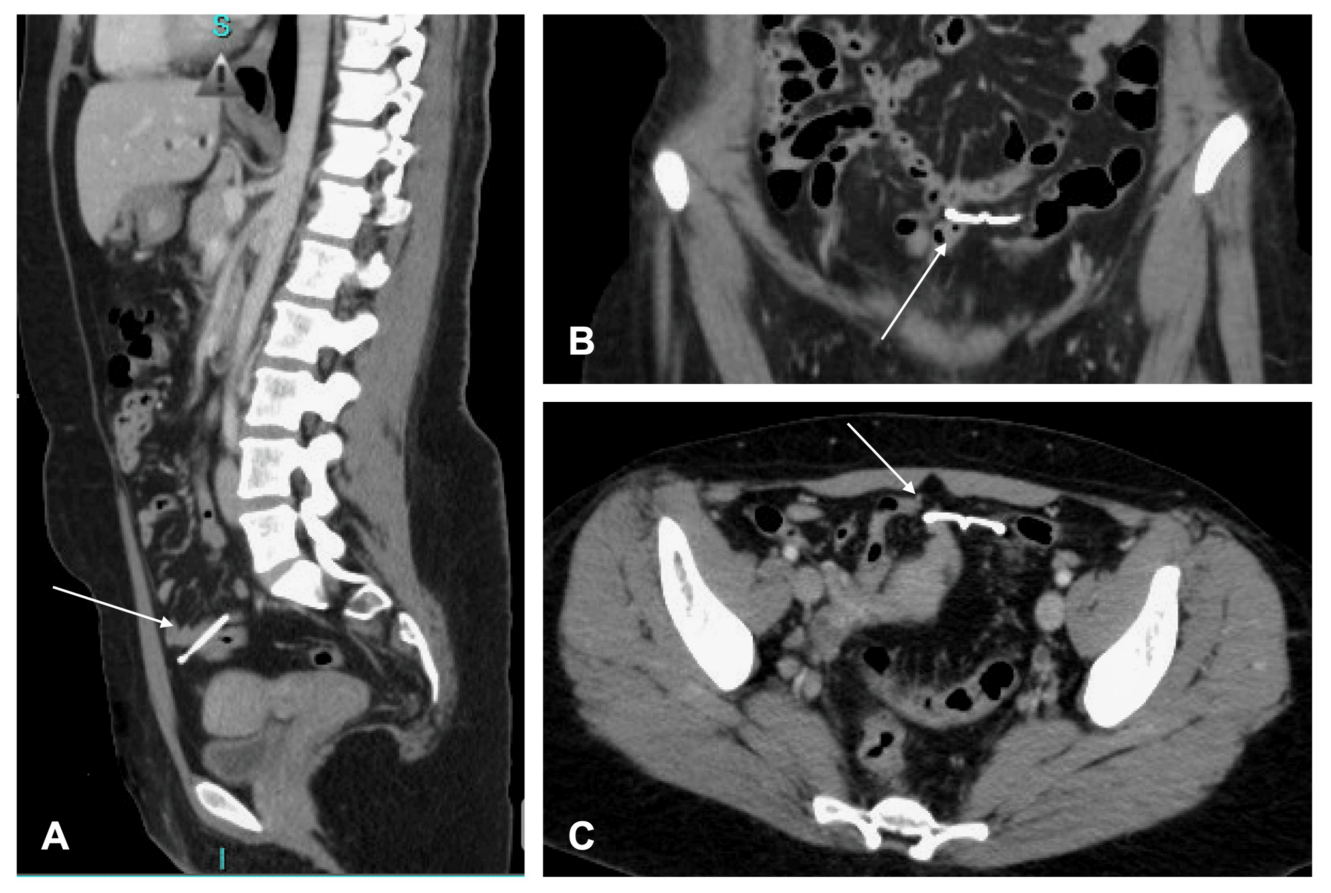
CT scan of the abdomen/pelvis, sagittal view (A), coronal view (B), and axial view (C), showing the intrauterine device (white arrow) in the inferior peritoneal cavity.
